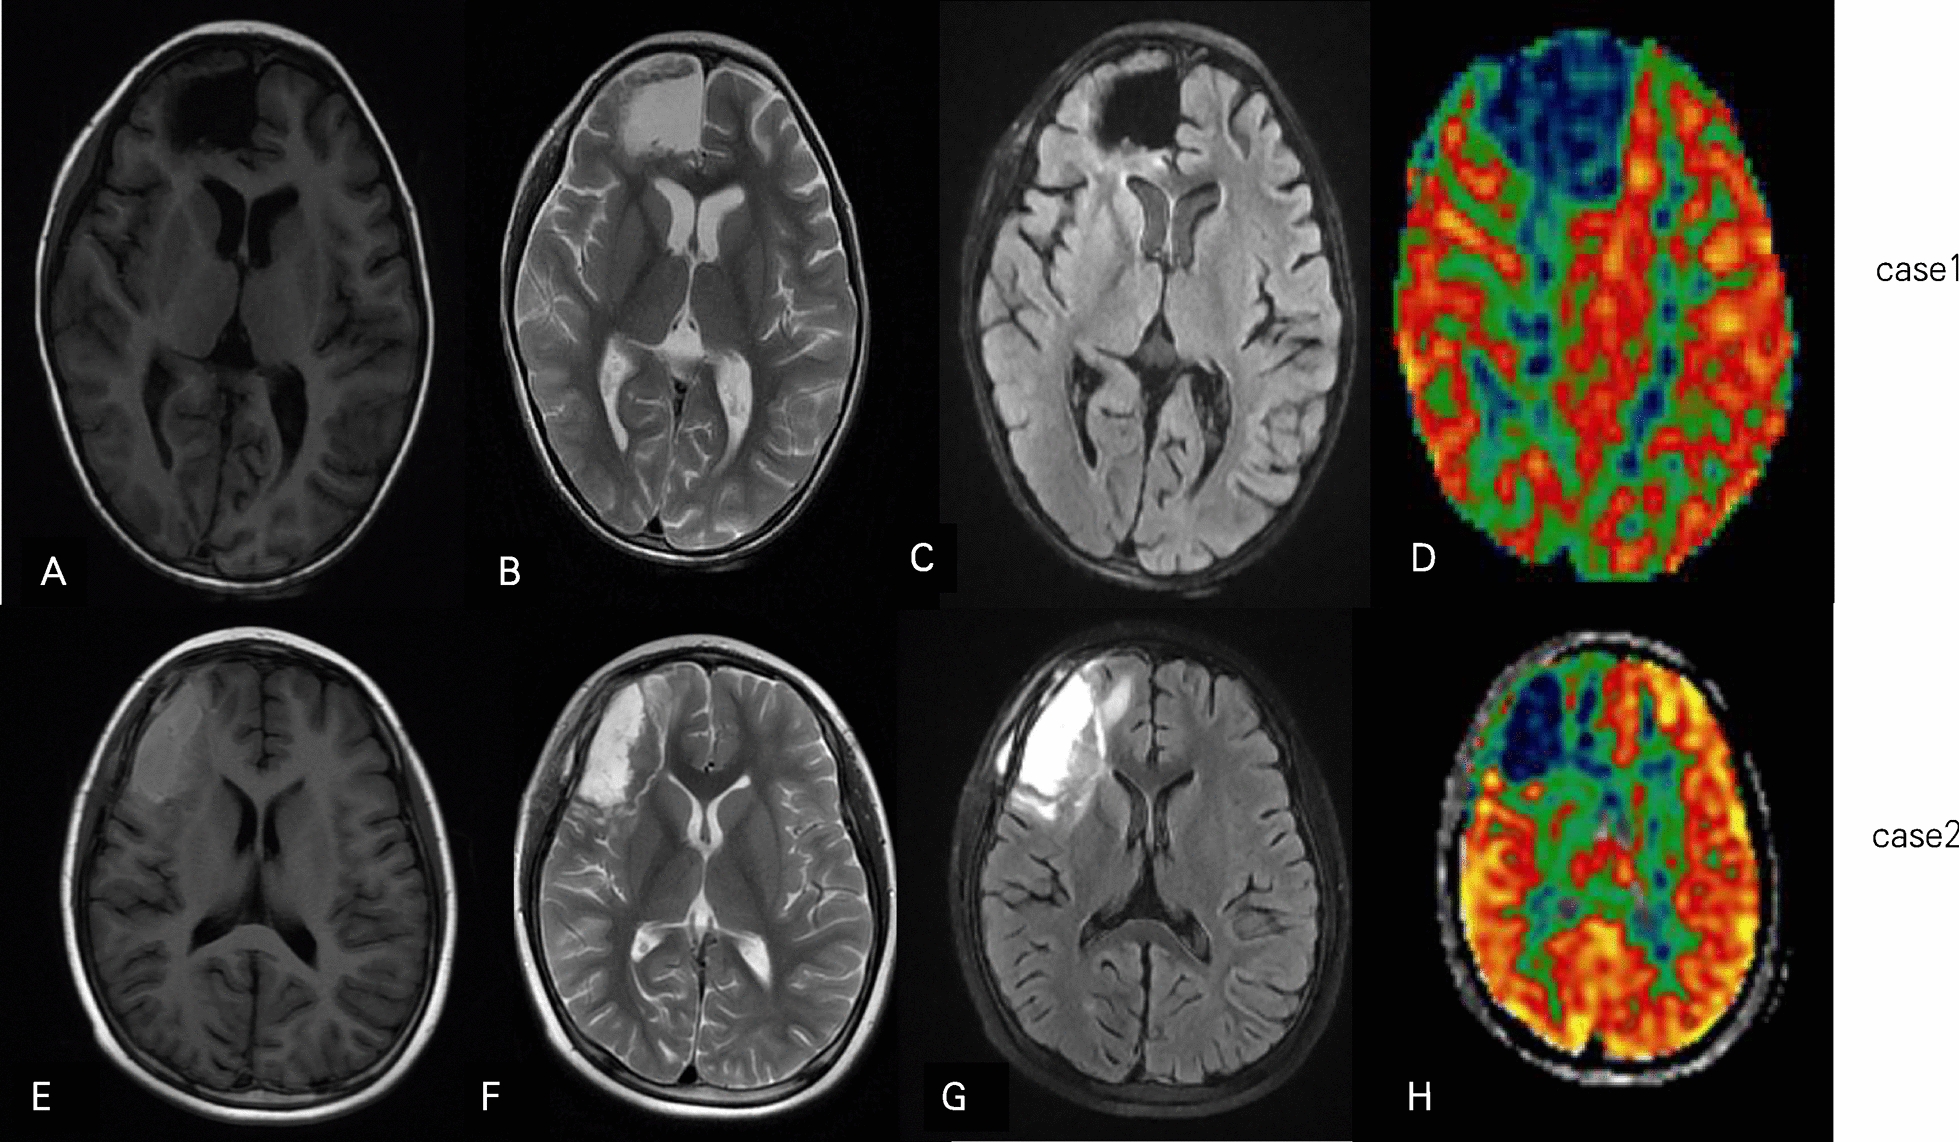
A–H Postoperative MRI and ASL imaging of both cases demonstrated that there were abnormally hypoperfusion areas in the right medial frontal lobe
